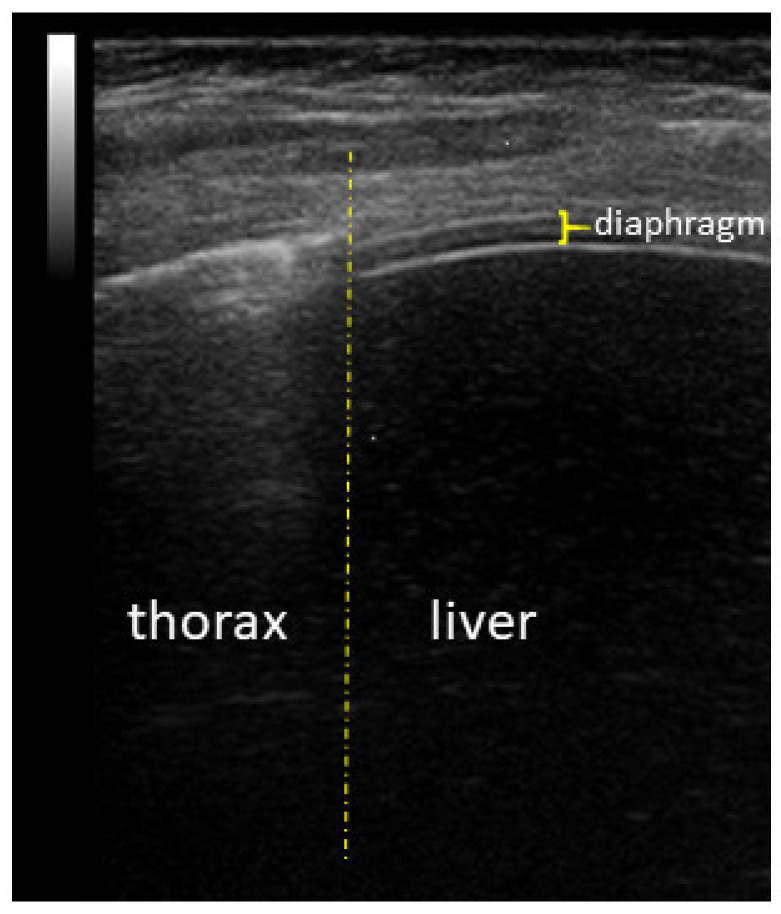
Ultrasound appearance of the diaphragm on the anterior axillar line.
